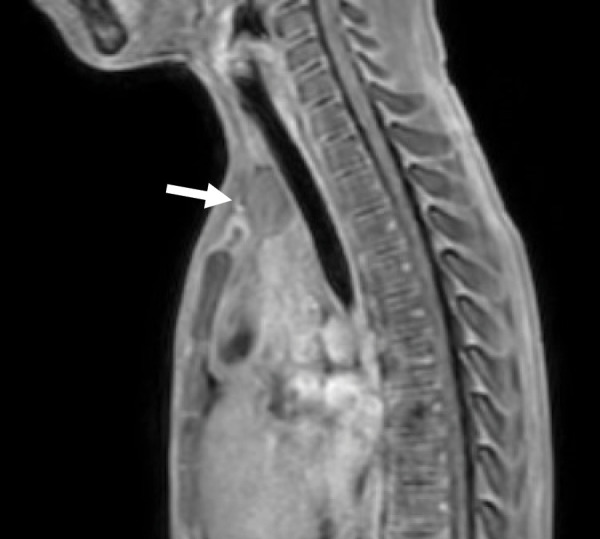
MRI. MRI showing cranial displacement of the thymus into the lower neck (white arrow). A solid cranial component adjacent to the teratomatous portion appears suspicious for malignant transformation.
